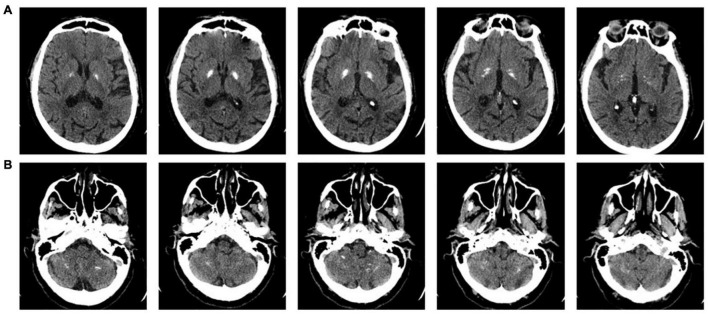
Axial sections of the present head computerized tomography (CT) scan of the patient. The upper row shows (A) the bilateral calcifications within the globus pallidus and cerebral white matter, and the lower row shows (B) the calcifications within the cerebellar hemispheres.
